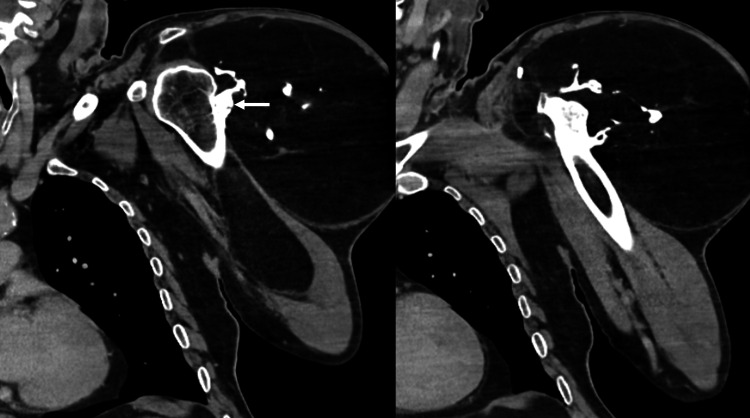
Coronal CT. A large lipomatous lesion adjacent to the left humerus is visible. An irregular osseous excrescence (arrow) is noted, lacking continuity with the humeral bone marrow. Additionally, multiple other foci of ossification within the lesion are observed.
